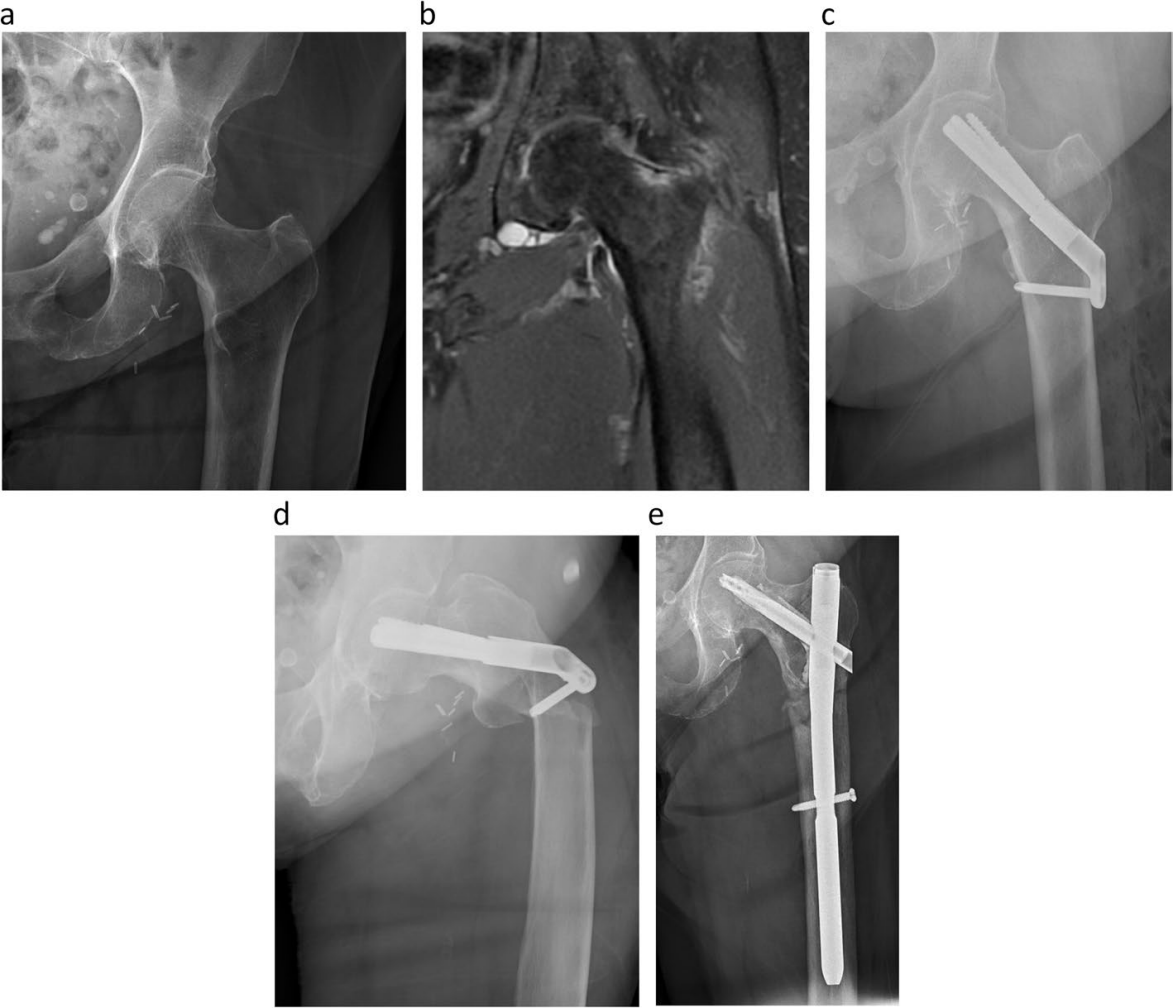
Panel a is an anteroposterior radiograph of the hip showing an incomplete nondisplaced fracture on the tension side of the femoral neck. Panel b is a coronal T2 fat suppressed MRI showing hyperintense signal on the tension side of the femoral neck. Panel c is an anteroposterior hip radiograph showing successful implantation of an FNS. Panel d is an anteroposterior hip radiograph showing peri-implant subtrochanteric femur fracture at the distal screw hole of the FNS. Panel e is an anteroposterior radiograph of the hip showing successful treatment with an intermediate cephalomedullary nail taken three months postoperatively
